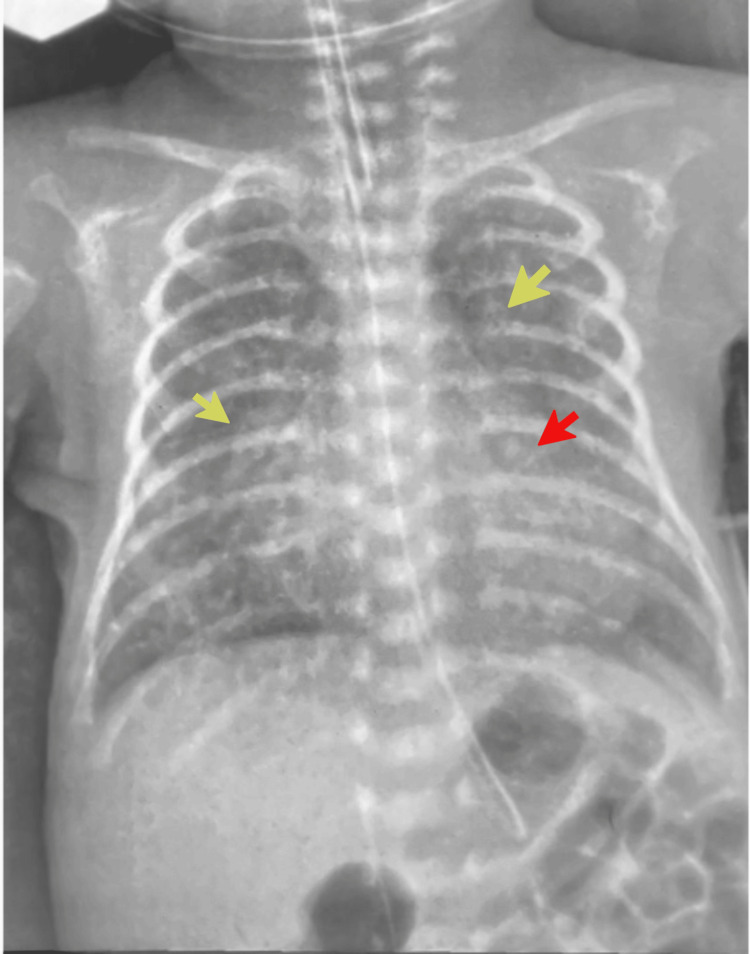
Pre-procedural chest radiography demonstrated marked cardiomegaly (red arrow) and profound pulmonary overcirculation, with bilateral diffuse opacities (yellow arrow) suggestive of pulmonary overcirculation.
